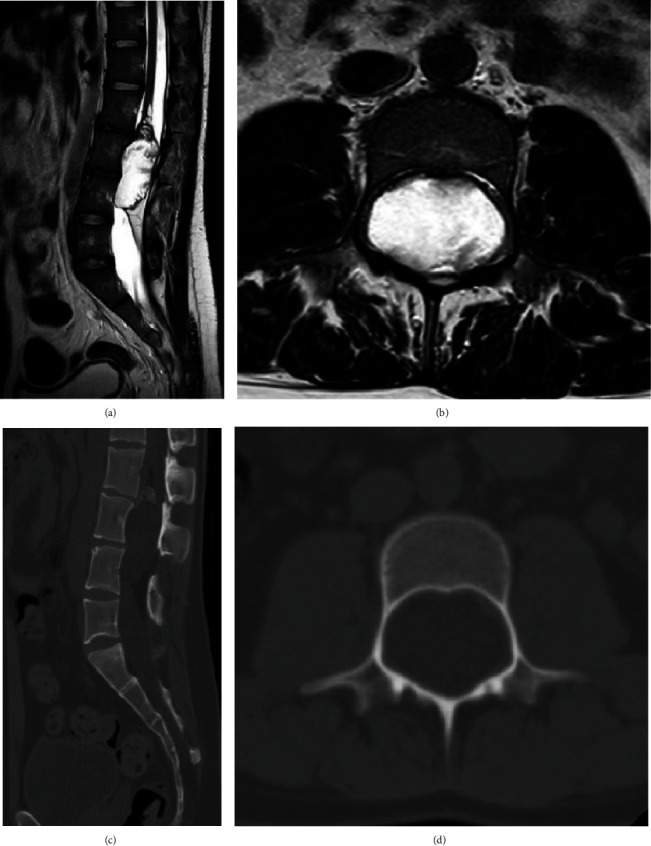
(a) Sagittal T2 MRI image showing hyperintense lesion with scalloping of the posterior aspect of the L3 vertebra. (b) Axial T2 MRI image showing heterogeneous hyperintense lesion causing expansion of the vertebral canal and narrowing of the pedicle bilaterally. (c) Midline sagittal CT image showing scalloping of the vertebra with lesions suggesting calcification at the top of the expansile lesion in the L3 level. (d) Axial CT image at the L3 vertebra level reinforcing the evidence of narrowing of the pedicles bilaterally, scalloping of the posterior vertebral body, and expansion of the vertebral canal.
